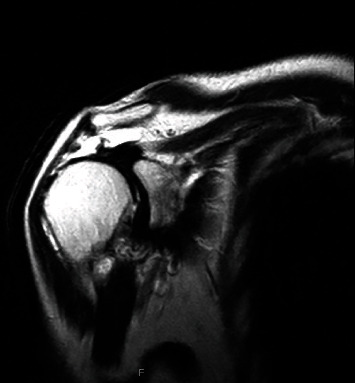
Coronal view of a T2-weighted MRI of the right shoulder following consultation for a rotator cuff tear.
